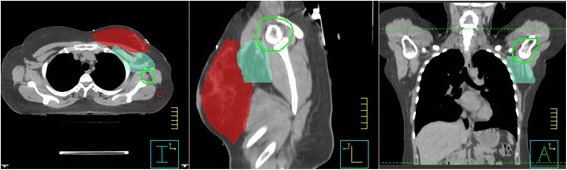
Axial, sagittal and coronal slices of the planning CT of patient 2. PTVp (primary) in *red*, PTVn (level I and II lymph nodes) in *turquoise* and humeral head + 10 mm in *green*
